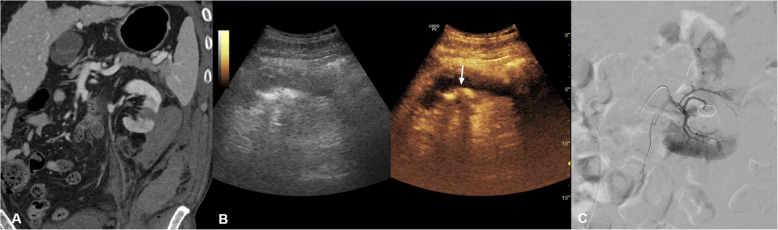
Renal arterial bleeding after partial nephrectomy. A Contrast-enhanced CT (portal venous phase, coronal image) demonstrates a postoperative hematoma. A subtle hyperattenuating focus is suspected; however, definite active extravasation is not confidently identified across phases. B CEUS demonstrates focal jet-like contrast extravasation adjacent to a segmental branch (arrow), supporting the decision to proceed with angiography for suspected active arterial bleeding. C Digital subtraction angiography demonstrates the bleeding focus (circled)
